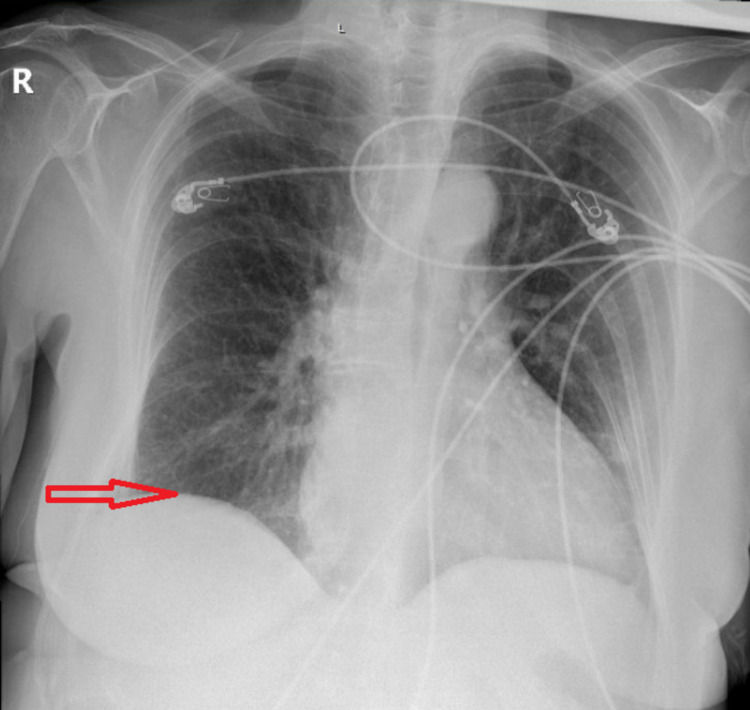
Portable chest X-ray anteroposterior (AP) view showing elevation of the right hemidiaphragm (arrow)
